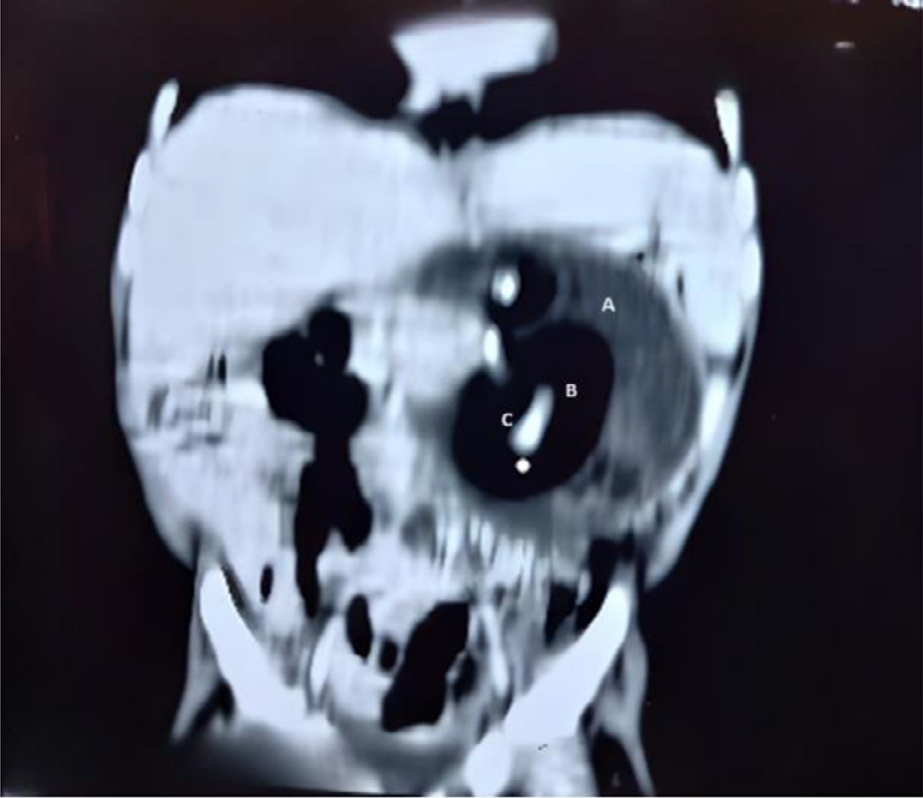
Coronal view of CT scan showing A (well-circumscribed heterogenous density mass in left mid abdomen and left lumbar region of 9.2 × 7.6 cm with smooth and sharp outline, having significant cystic (A), fat (B), and calcified component (C).
